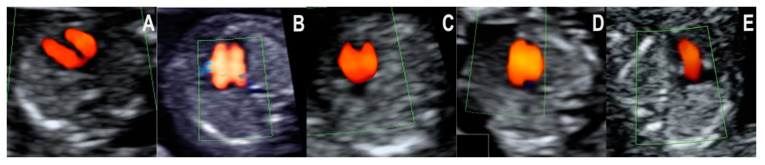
Color doppler flow patterns encountered in the four-chamber view. (A): Normal filling of both ventricles. (B): Filling of both ventricles, but the cardiac axis is abnormally shifted to the left. (C): Common inflow into the ventricles. (D): Filling of both ventricles, but the left ventricle appears smaller. (E): Filling of only one ventricle.
